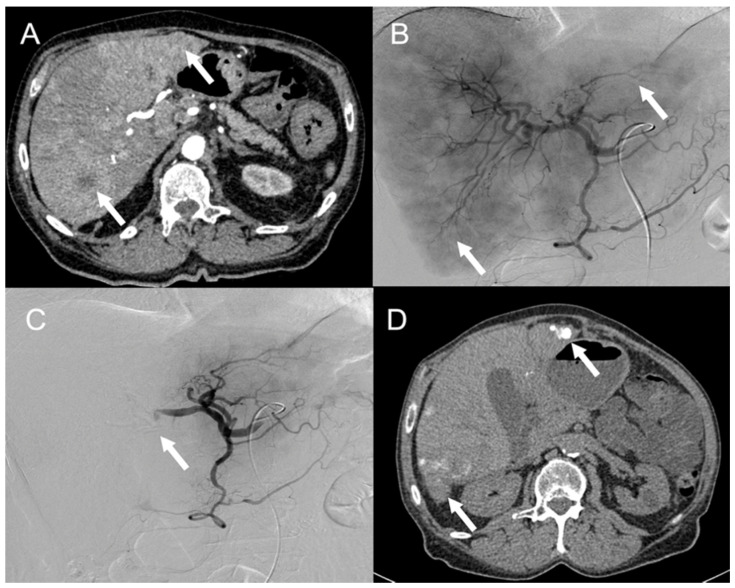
A 66-year-old patient with exclusively hepatic metastasis of a neuroendocrine tumor of unknown origin presented with important abdominal pain and carcinoid syndrome despite cold somatostatin analogues treatment. Preembolization contrast-enhanced arterial CT (A) and digital subtraction angiography (DSA) (B) show bilateral hypervascular liver metastases (arrows). DSA performed after a first TACE session in the right liver (C) shows complete devascularization of right liver lesions and contrast stagnation in the right branch of the hepatic artery (arrow). Postembolization CT performed 2 months after a second TACE session (D) performed on the left liver shows persistent Lipiodol retention in the treated metastasis (arrows).
